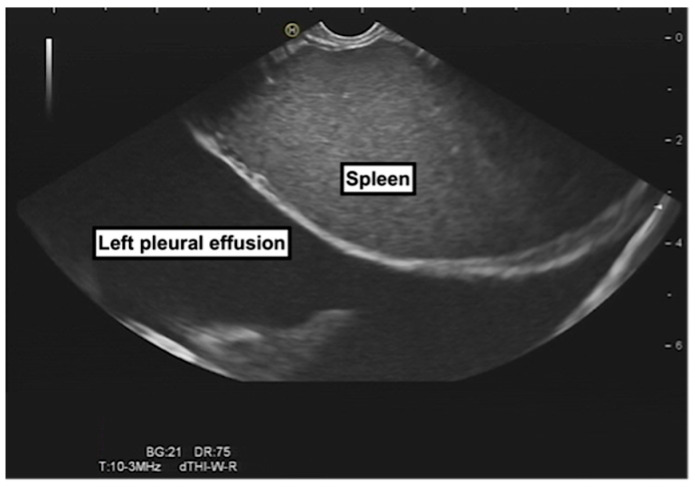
Endosonographic ultrasound demonstrated a moderate left pleural effusion.
